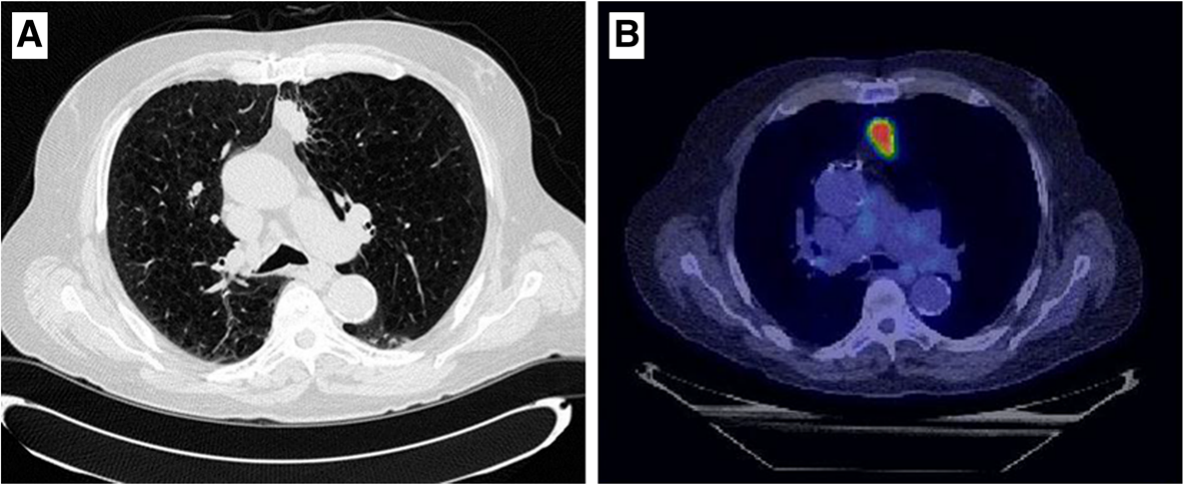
Chest CT and PET scan of a 70 year-old male revealed a 3.3 cm hypermetabolic mass with an SUV maximum of 12.3.
